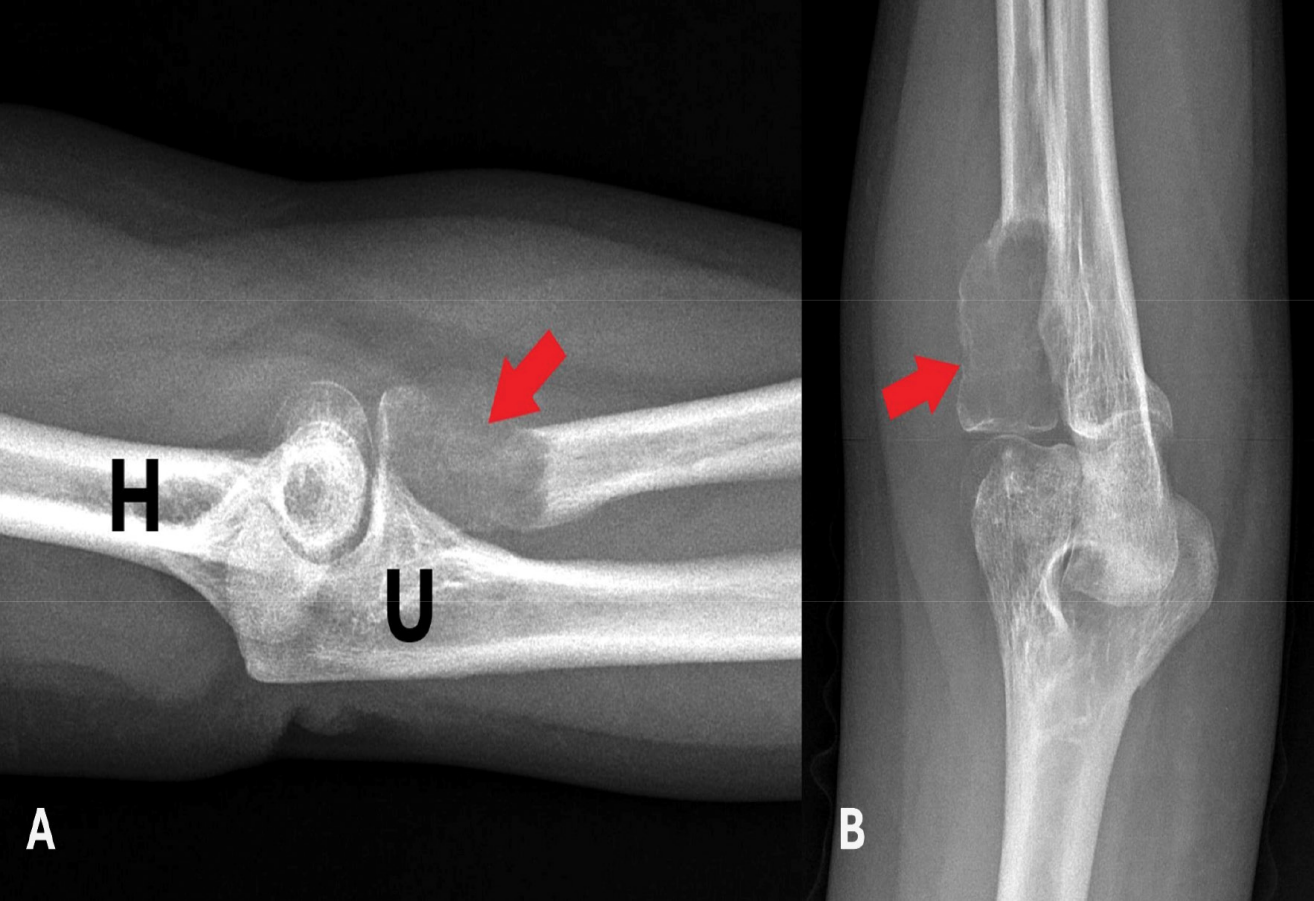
A‑B On plain radiography, a well‑defined, expansile, lytic bone lesion with thin internal septations is present in the radial head (indicated by red arrows). Adjacent osseous structures are labeled as humerus (H) and ulna (U).
